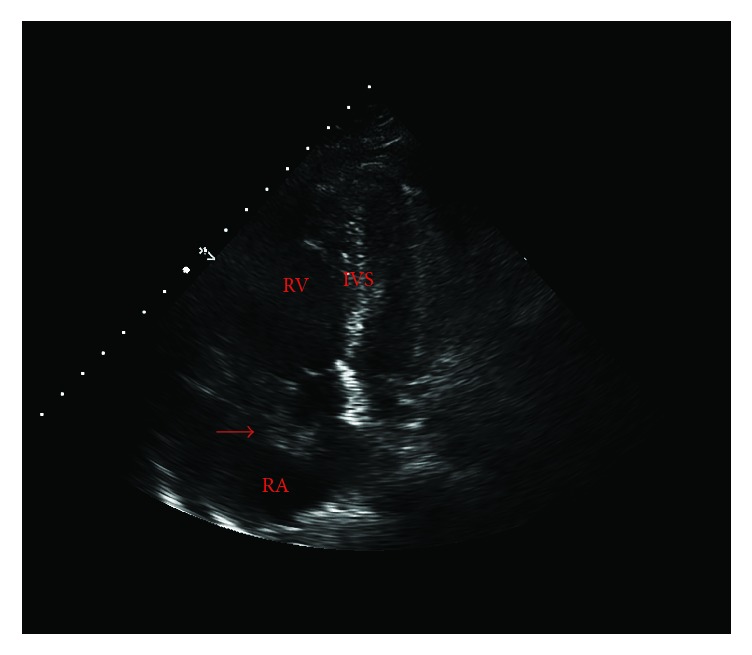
Intraoperative transthoracic echocardiography, 4 chamber apical view showing a right atrial thrombus (arrow), right ventricular dilatation, right atrial dilatation, and septal bowing. RV, right ventricle; RA, right atrium; IVS, interventricular septum; LA, left atrium; LV, left ventricle.
